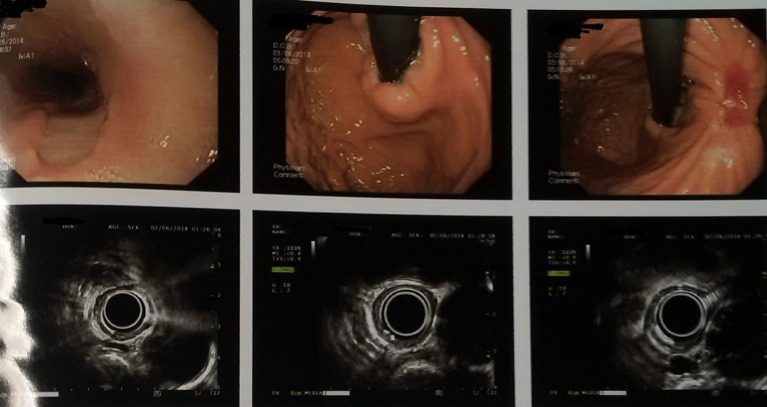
Endoscopic ultrasonography images (post chemotherapy).
